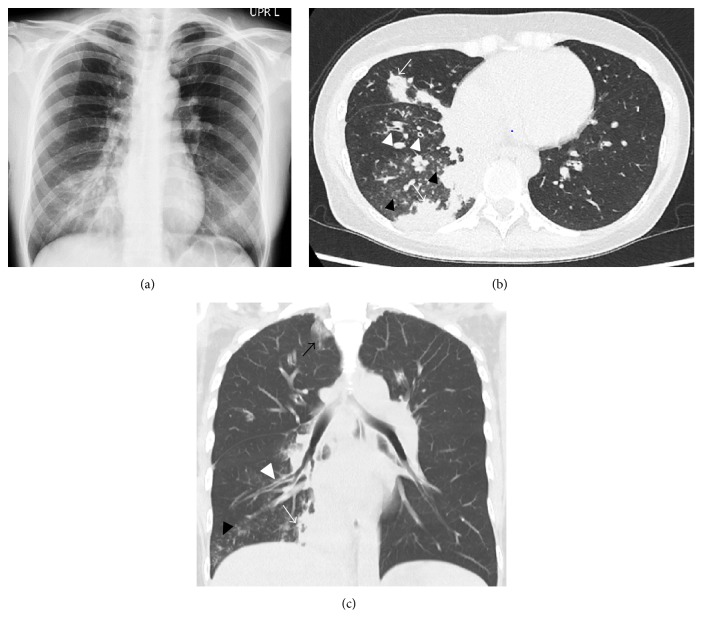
Chest radiography (a), axial view (b), and coronal view (c) of chest computed tomography (CT) images of the case. The chest X-ray showed a high-density area in right lower lobe. The chest CT revealed airspace consolidations (white arrows), thickening of bronchial walls (white triangles), and numerous centrilobular nodules (black triangles). The coronal view showed ground-glass opacity in the right upper lobe (black arrow).
